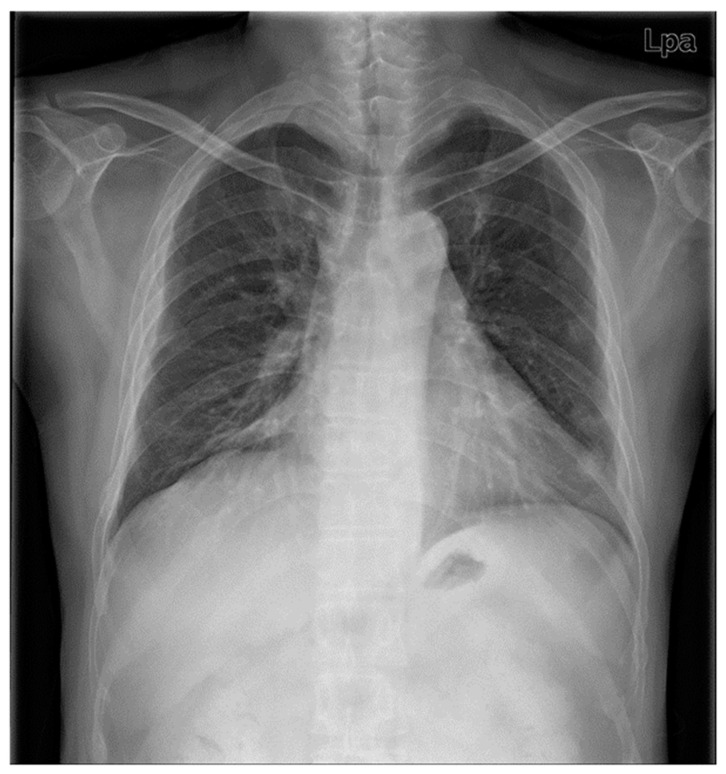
Preoperative chest radiography shows diffuse peribronchial cuffing in both lungs, with marked narrowing trachea, suggesting known relapsing polychondrits, and healed fracture at Rt. 8,9th and Lt. 8th rib.
